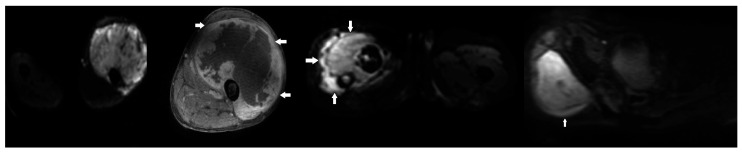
DWI scan: —tumor margin infiltration.
